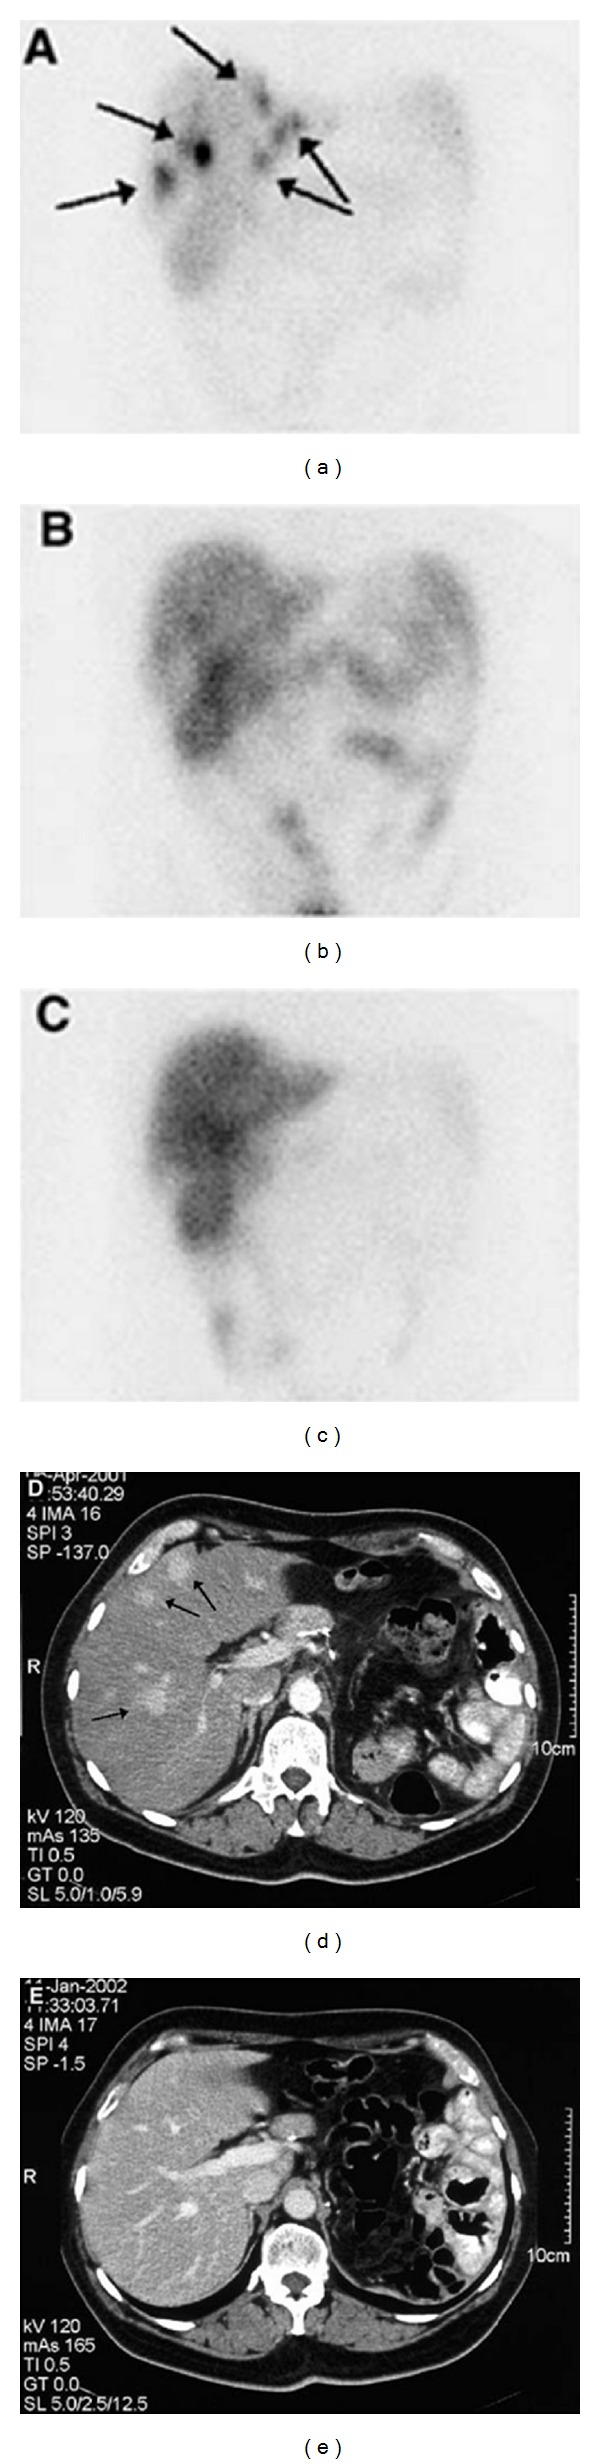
(a)–(c) Planar scans of the abdomen, 3 days after the injection of 200 mCi ^177^Lu-octreotate in a patient with liver metastases of an operated neuroendocrine pancreatic tumor. (a) After the first treatment; (b) after the second treatment; (c) after the fourth treatment. Note the loss of intensity of uptake in the liver lesions (arrows in (a)). This sign virtually always indicates a tumor volume response. (d) and (e). CT scans of the same patient: (d) before treatment; (e) 3 months after the last treatment. Tumor (arrows in (d)) is not demonstrated on (e). Neither MRI nor octreoscan could demonstrate definite tumor deposits at that time [66].
